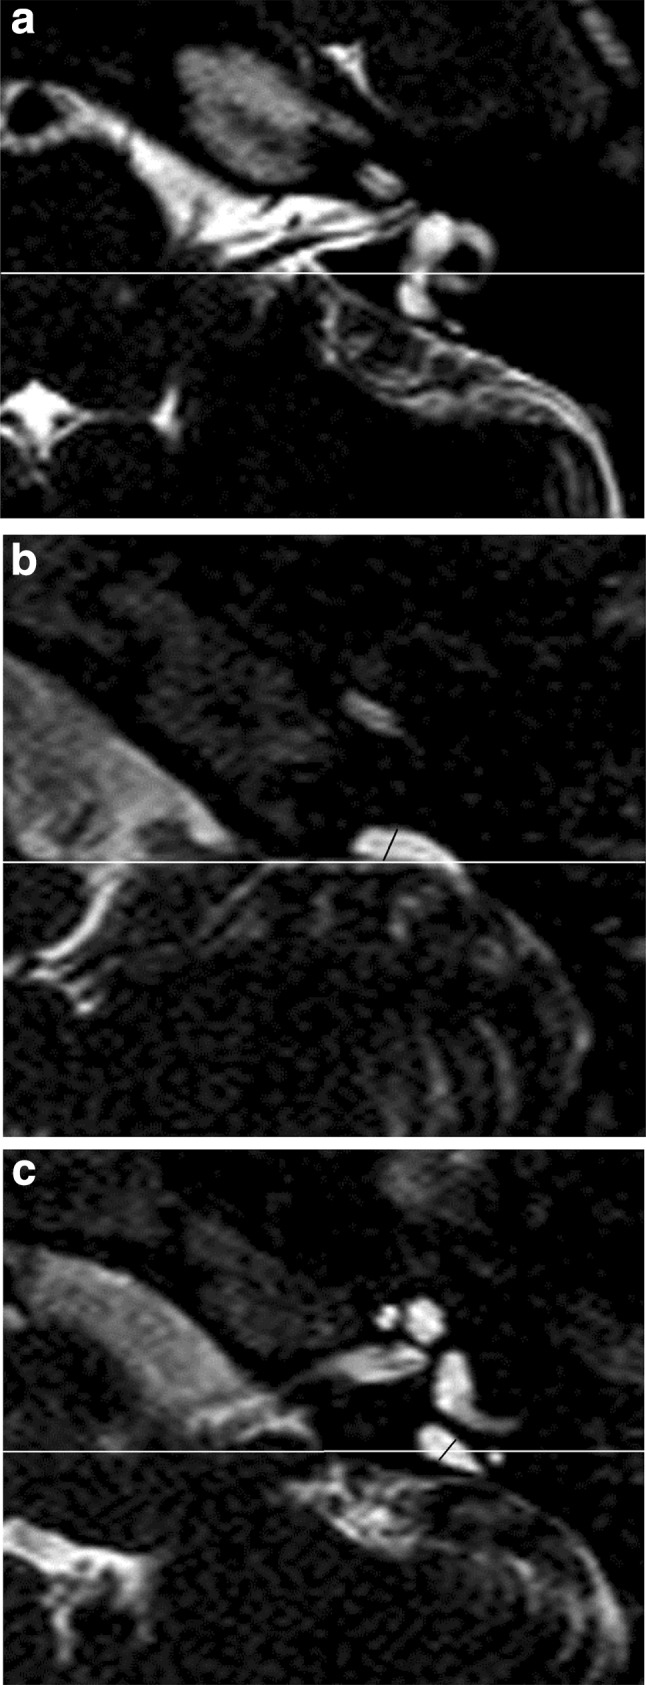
Intra-osseous measurement methodology. T2 DRIVE axial MR images demonstrating the vestibular, opercular and midpoint planes in a patient with bilateral large endolymphatic sac anomaly but no septations. a White line corresponds to the vestibular plane defined by the horizontal plane at the level of the dorsal common crus as it arises from the vestibule. b White line corresponds to the opercular plane defined by the horizontal plane at the level of the superior opercular lip. Black line corresponds to the opercular measurement. c White line corresponds to the midpoint plane, defined as halfway anteroposteriorly between the vestibular and opercular planes. Black line corresponds to the mid-point measurement
